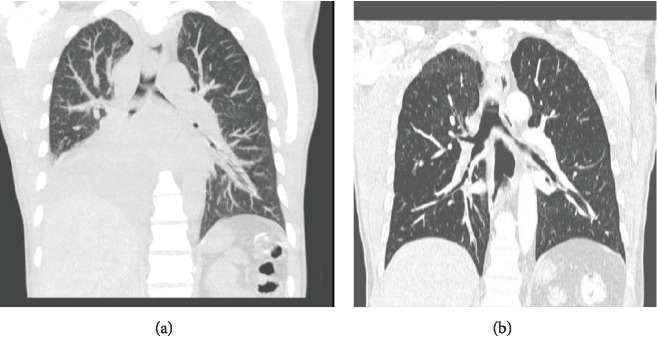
(a) Chest CT without contrast prior to chest radiation showing a 5.8 × 4.5 cm right infrahilar mass obstructing the bronchus intermedius with right paratracheal adenopathy. Postobstructive consolidation of the right lower lobe and patchy infiltration of the right middle lobe. (b) Chest CT with IV contrast postchest radiation revealing significant improvement in the right hilar lesion. There is slight thickening around the right main bronchus; the large soft tissue lesion has almost totally resolved with resolution of postobstructive changes.
